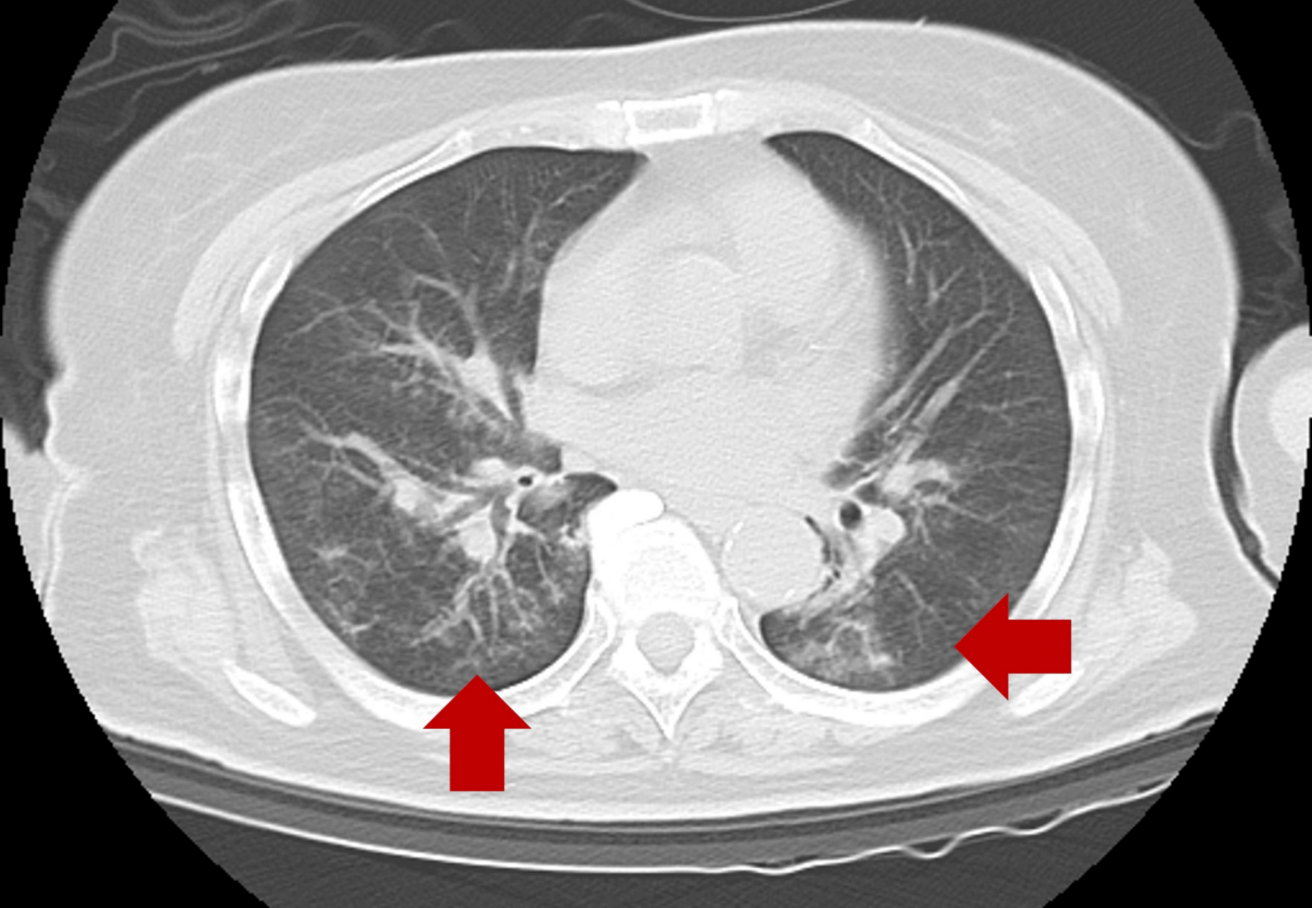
Chest Computed Tomography on Arrival The area indicated by the arrows demonstrates a pulmonary infiltrate consistent with pneumonia.
